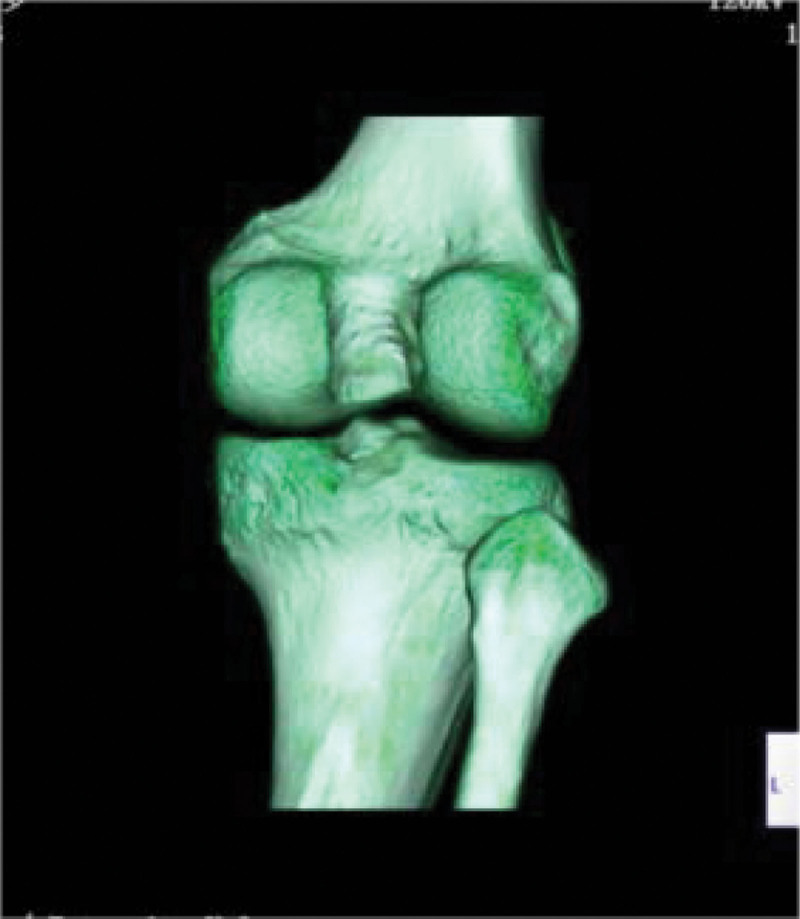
Preoperative three-dimensional CT image. CT = computed tomography.
